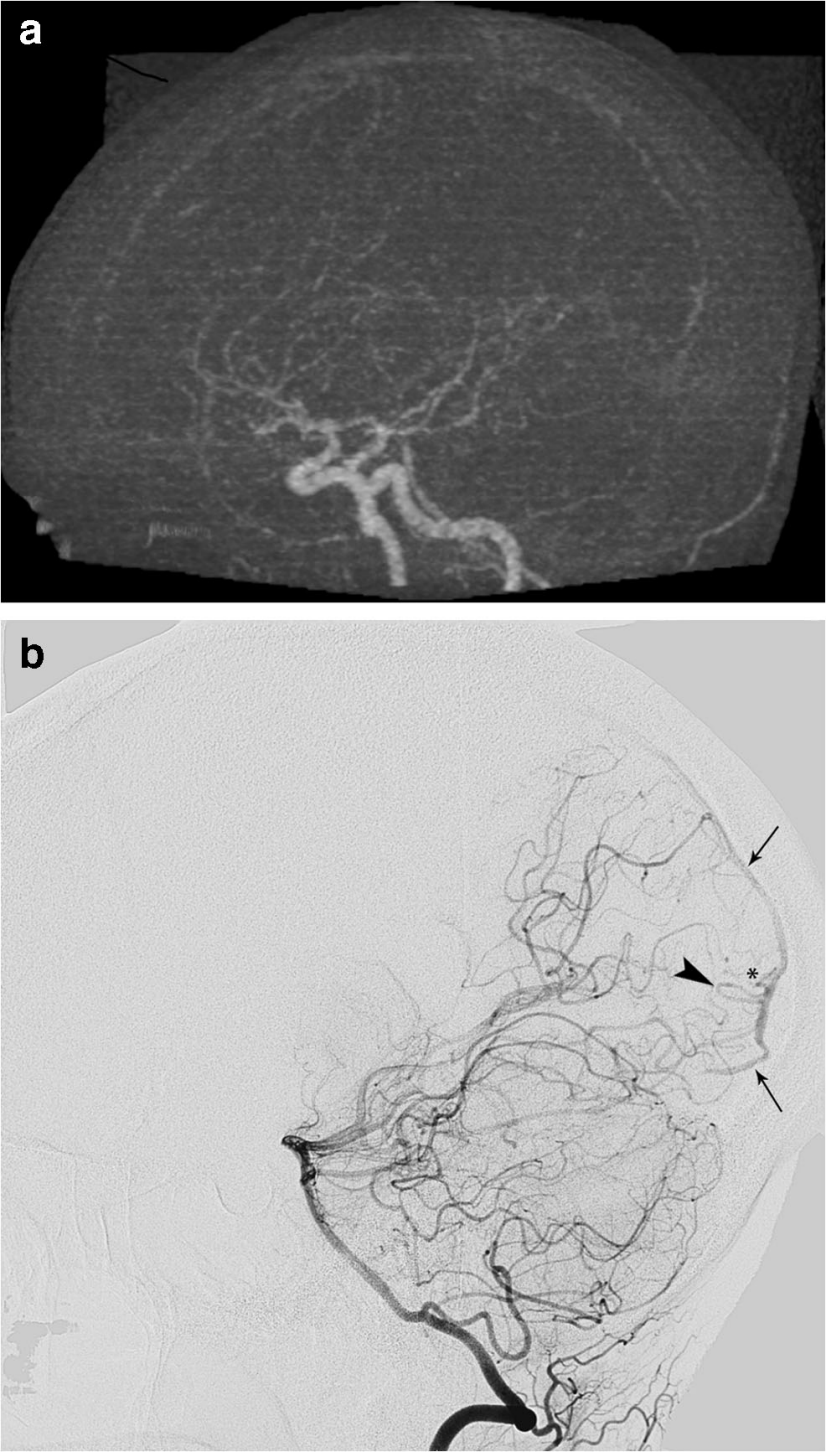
Panels a and b (left vertebral artery injection) are from the case where a false-negative 4D-CTA missed a micro-AVM. The DSA shows early venous filling (arrows). The arrowhead and asterisk respectively depict a feeding artery and the area of the shunt
